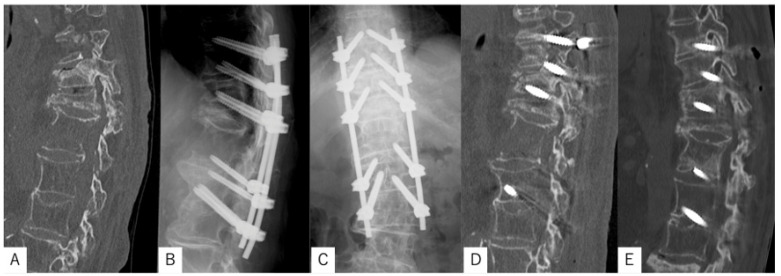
Spinal fracture case with DISH involving an 89-year-old woman. (A) Preoperative CT showing a marked anterior distraction gap (17 mm) at L1. (B) Postoperative posteroanterior radiogram. (C) Postoperative lateral radiogram. Posterior fixation was performed from Th10 to L3 (five levels) using TSDs. (D) CT 1 week postoperatively showing a residual anterior gap of 17 mm. (E) CT 9 months postoperatively showing complete closure of the gap (0 mm), with sufficient bone bridging and union and no implant loosening.
